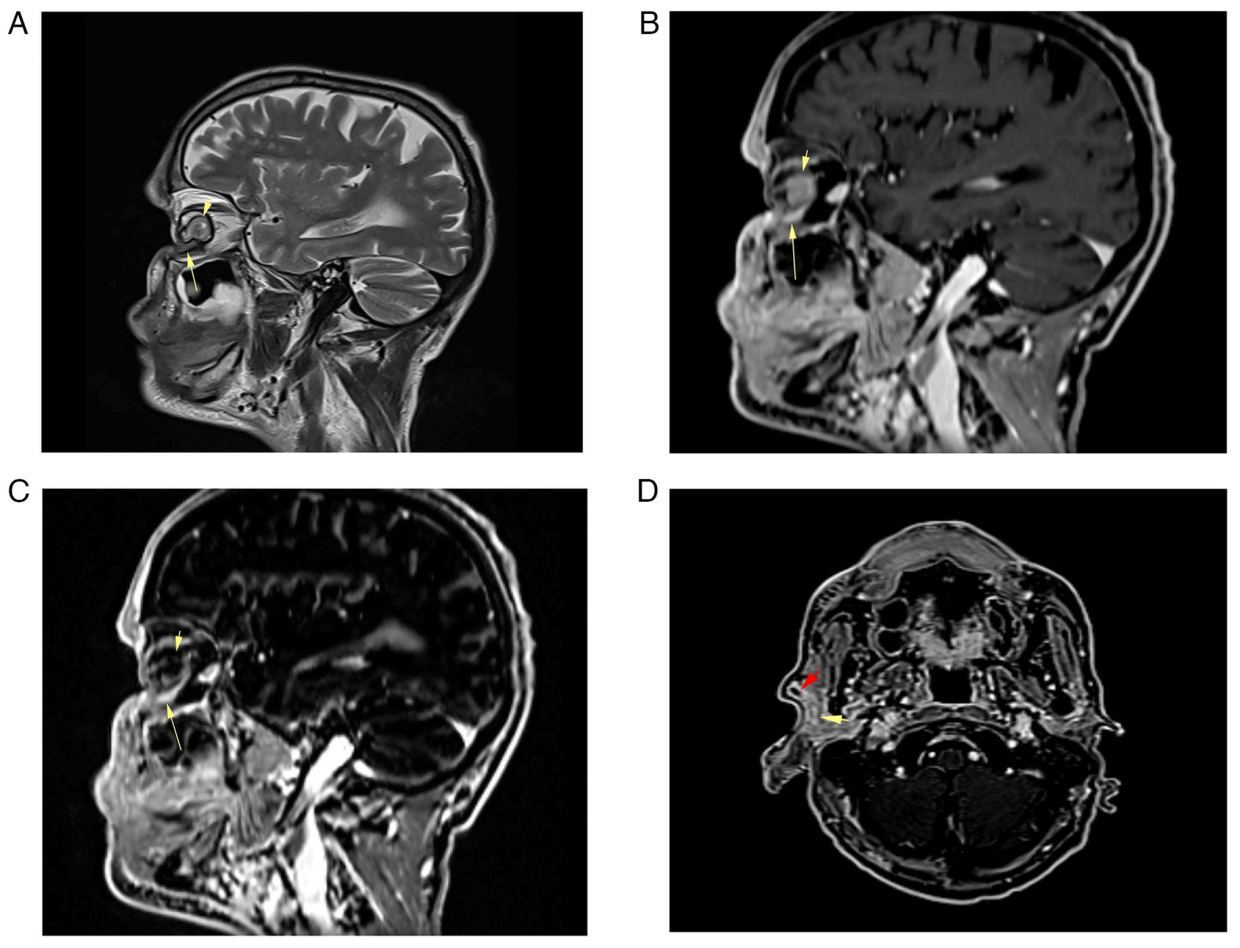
Post-treatment magnetic resonance imaging showing a significant reduction (~90%) in the tumor size. (A) A sagittal T2-weighted image showing a shrunken intraorbital mass (arrow) with atrophy of the right orbit, resulting in phthisis bulbi, which contained a hemorrhagic component (arrowhead). (B) A sagittal post-contrast fat-suppression T1-weighted image showing an infraorbital linear enhancing component measuring 8×4 mm, with the intraorbital homogenous component appearing hyperintense (arrowhead). (C) A sagittal subtraction sequence showing an enhancing infraorbital linear focus (arrow), representing residual tumor after chemotherapy, with the intraorbital hemorrhagic component showing no post-contrast enhancement (arrowhead). (D) Post-treatment axial fat-suppressed T1-weighted images demonstrated a significant reduction in the size of both lymph nodes (yellow and red arrows).
